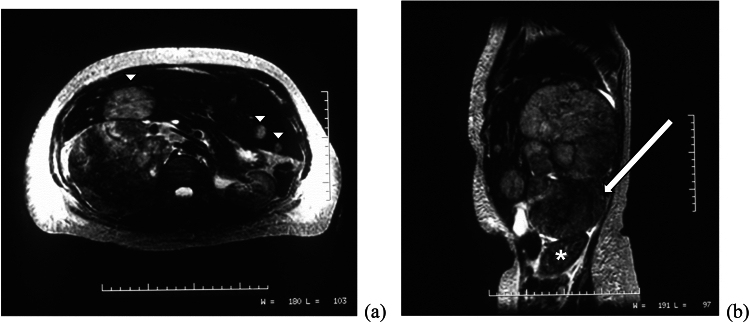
A 60-year-old woman presented with marked hirsutism and elevated androgen levels. Axial (a) and sagittal (b) T2-weighted MRI images demonstrate a large right adrenal mass (arrow) which displaces the kidney (*) inferiorly. Multiple liver metastases are also present (arrowheads)
